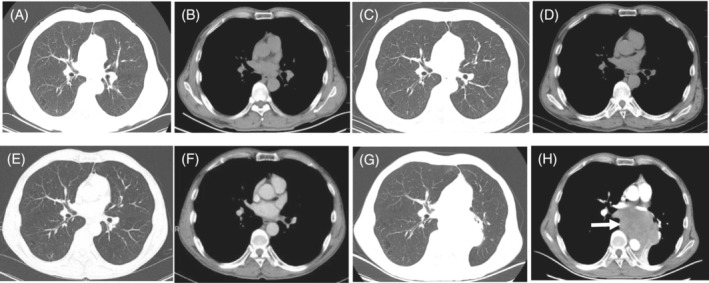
Dynamic changes in chest CT during admission and follow‐up. Chest CT at the time of diagnosis with LEMS (A, B). 3 months (C, D), 6 months (E, F) after the diagnosis of LEMS. Chest CT (G) and enhanced chest CT (H) 10 months after the diagnosis of LEMS demonstrated lung cancer of posterior inferior mediastinum and left pulmonary hilum (as indicated by the arrow).
